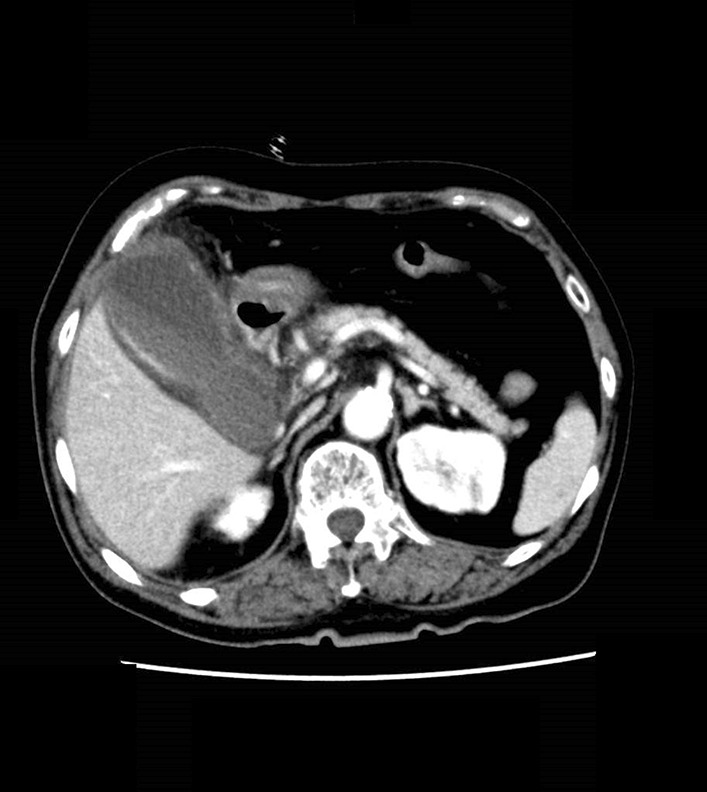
Contrast enhancement computed tomography
